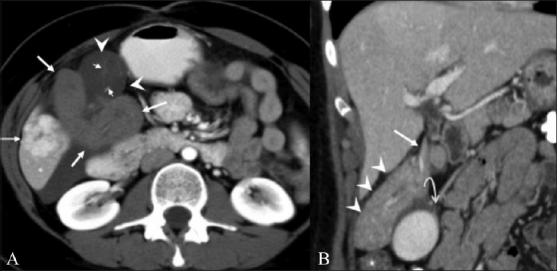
Axial contrast-enhanced CT scan (A) shows torsion of the accessory lobe (arrows), with reduced enhancement compared to the surrounding liver. The gallbladder (GB) appears adherent to the twisted lobe, is anteriorly rotated (arrowheads), and shows wall edema and faint mucosa (small arrows). Focal nodular hyperplasia is incidentally noted (curved arrow). Coronal, reformatted CT scan (B) shows the thin pedicle (arrow) of the accessory liver lobe (arrowheads). Notice the decrease in the GB wall edema (curved arrow) and improved enhancement of the accessory lobe, indicative of interval detorsion
